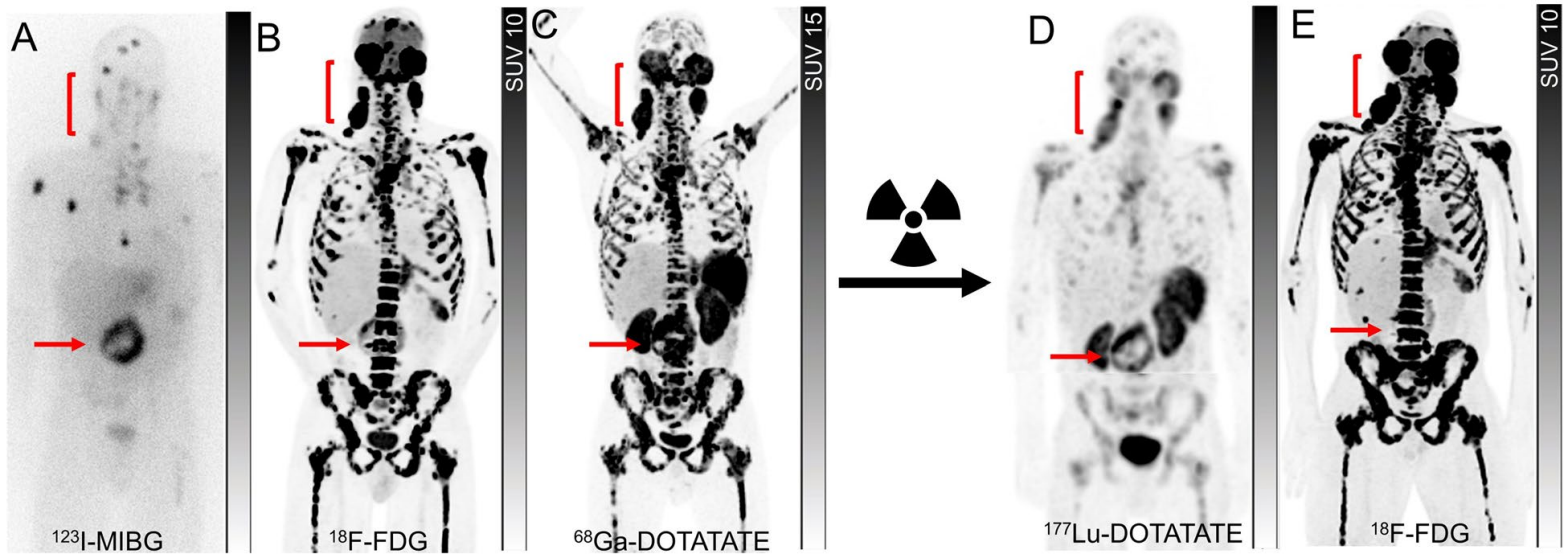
A patient with the organ of Zuckerkandl metastatic paraganglioma with progression on three lines of chemotherapy, three lines of targeted therapies and immunotherapy was referred for radionuclide therapy. ^123^I-MIBG planar imaging (A) showed intense uptake in the organ of Zuckerkandl (arrows) and a limited number of bone metastases compared to far more extensive bone metastases and lymphadenopathy in the bilateral posterior neck (brackets) on a contemporaneous ^18^F-FDG PET (B). Therefore, the patient was not considered suitable for treatment with ^131^I-MIBG. ^68^Ga-DOTATATE PET (C) showed high tracer avidity (Krenning score 4) in most sites of disease similar in distribution to ^18^F-FDG PET, hence the patient was deemed suitable for ^177^Lu-DOTATATE. The post-treatment ^177^Lu-DOTATATE SPECT (D) showed moderate to intense uptake in the primary site and lymphadenopathy while the vast majority of bone metastases retain only mild to moderate tracer uptake (Krenning score 2–3). The patient developed progressive pancytopenia, which combined with the low post-treatment radiotracer uptake, did not advance with further cycles of ^177^Lu-DOTATATE. ^18^F-FDG PET (E) 2 months post-^177^Lu-DOTATATE showed further progression of the disease
